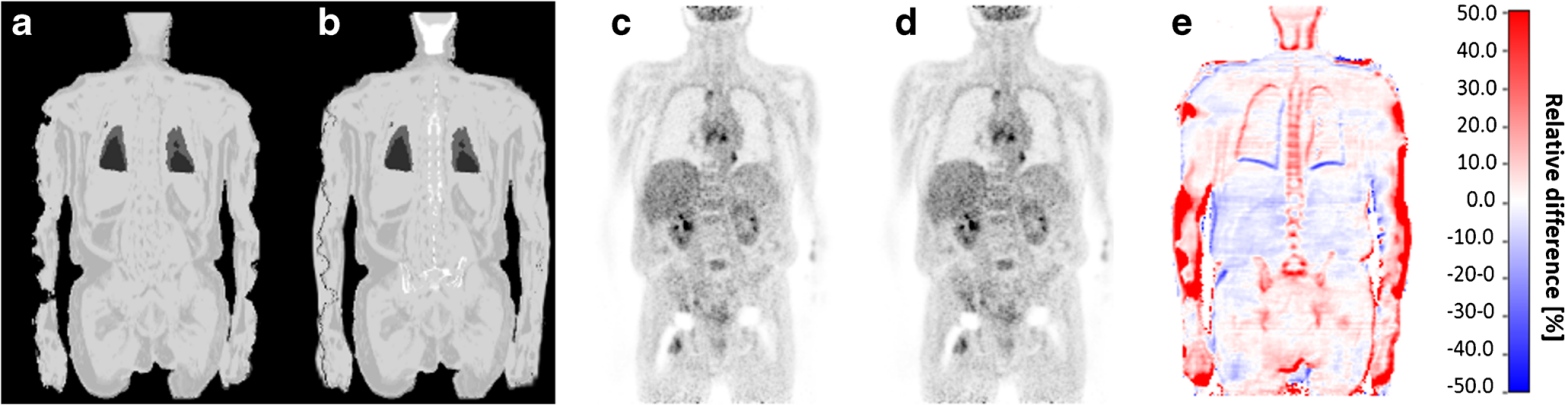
Patient example with injected ^18^F-FDG: standard 4-compartment μ-map serving as reference (a) and improved 6-compartment μ-map (b). c and d show corresponding attenuation-corrected PET data ((c) 4 compartments, (d) 6 compartments). c and d show no visible difference. e shows a calculated relative PET difference map, highlighting differences with color. The colored bar in (e) provides the quantitative bias of relative undercorrection (red) or overcorrection (blue) when comparing PET data corrected with 6-compartment μ-map to PET data corrected with a 4-compartment μ-map (reference). Maximal relative differences are observable in the previously truncated areas along the arms and in areas where bone was added to the μ-map
